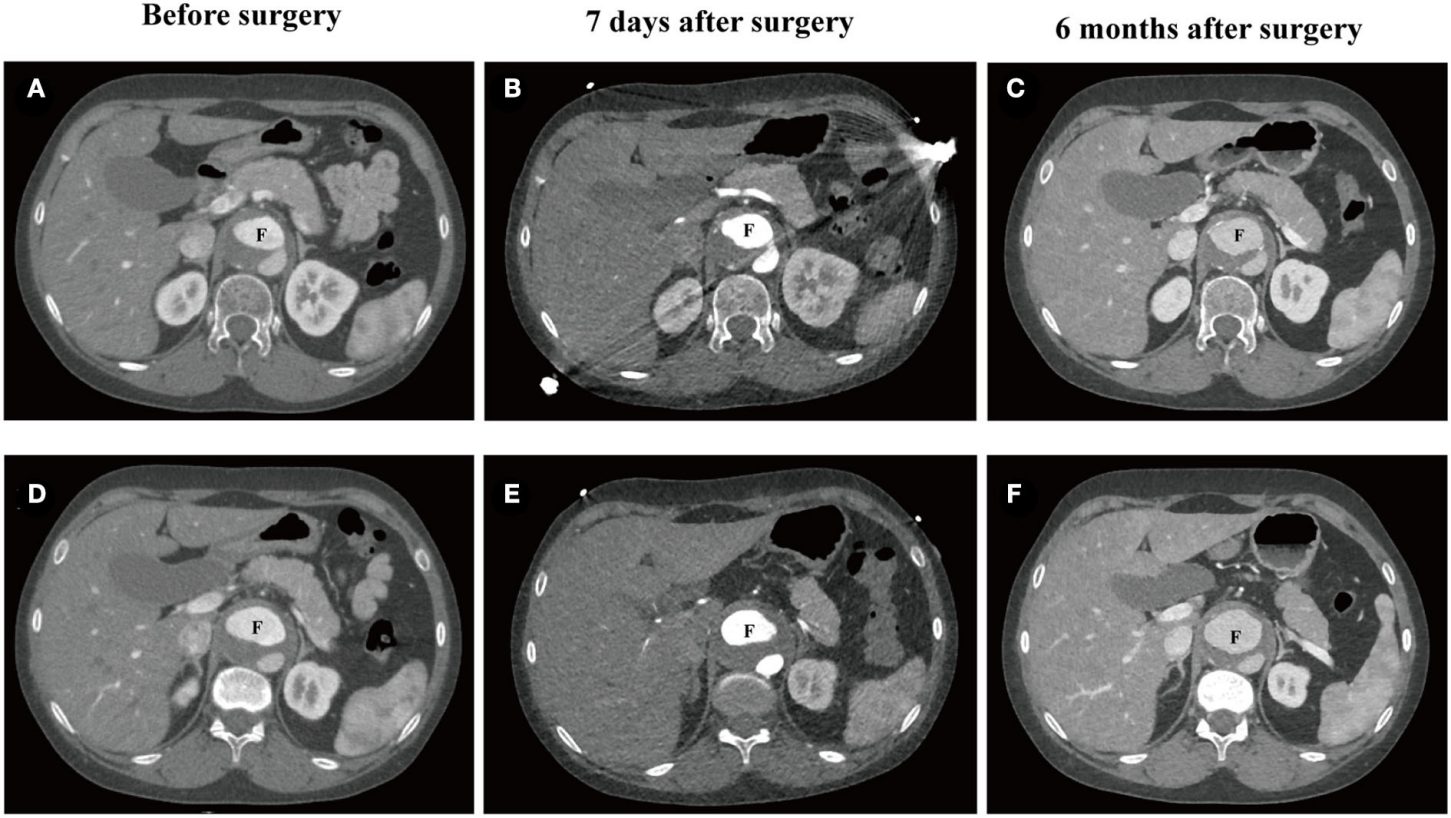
CTA of abdominal aortic dissection in the 25-year-old female patient. (A–C) The false lumen at the position of intimal tears before surgery (32.8 × 22.8 mm^2^), 7 days after surgery (32.6 × 22.2 mm^2^), and 6 months after surgery (35.9 × 26.8 mm^2^). (D–F) Maximum diameter of the false lumen of AAD before surgery (38.9 × 23.1 mm^2^), 7 days after surgery (39.3 × 24.6mm^2^), and 6 months after surgery (40.9 × 29.1 mm^2^). CTA, computed tomography angiography; F, false lumen.
